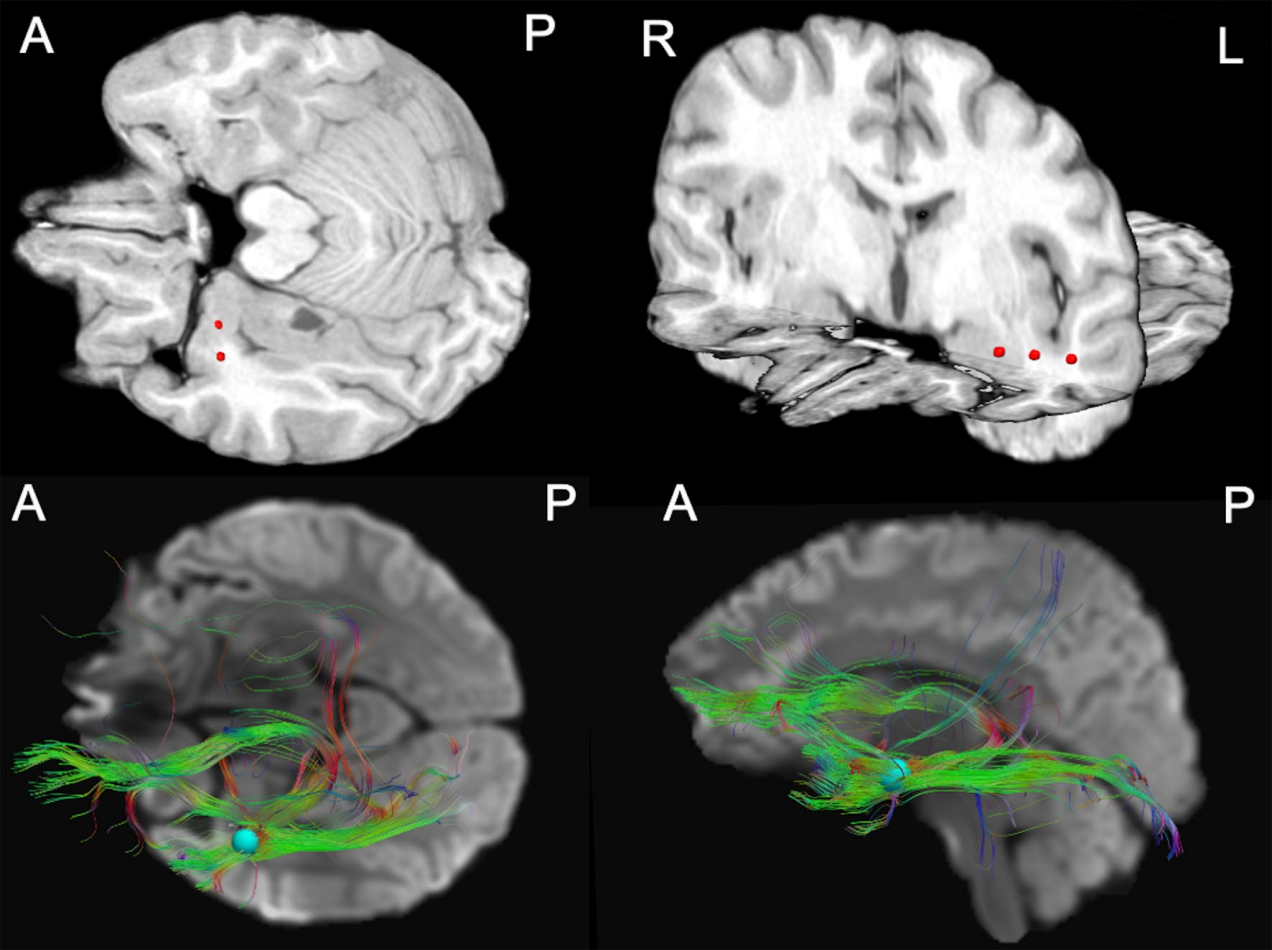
Tractography results. The upper row shows the location of the electrodes which were associated with unilateral blinking in one representative patient (ID3). The location of the stereo-EEG electrodes was determined by a co-registration of the preoperative MRI and postoperative CT scan.The lower row shows the HARDI fiber tracking results seeding from a 5 mm big sphere. The spheres were positioned around one of the electrodes shown in the 2D images above. For reasons of clearness, only 10% of the reconstructed fibers are shown. As background, average diffusion weighted imaging maps were used. *A* anterior, *L* left, *P* posterior, *R* right
